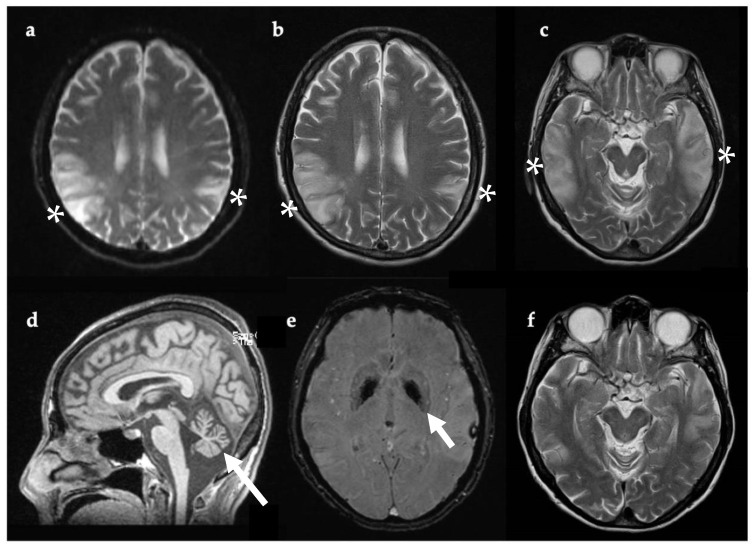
Axial (a–c,e,f) and sagittal (d) brain MRI, 11 days after symptom onset (a–e) and one year later (f). Bilateral temporoparietal cortical and gyral swelling shown with white asterix in (a), DWI (b), and (c) T2w. Evidence of cerebellar atrophy (d, white arrow). SWI sequence showing pallidal mineralization (e, white arrow). MRI one year later (f) showed regression of lesions previously evident (c). Abbreviations: DWI, diffusion-weighted imaging; SWI, susceptibility-weighted imaging; T2w, T2-weighted imaging.
